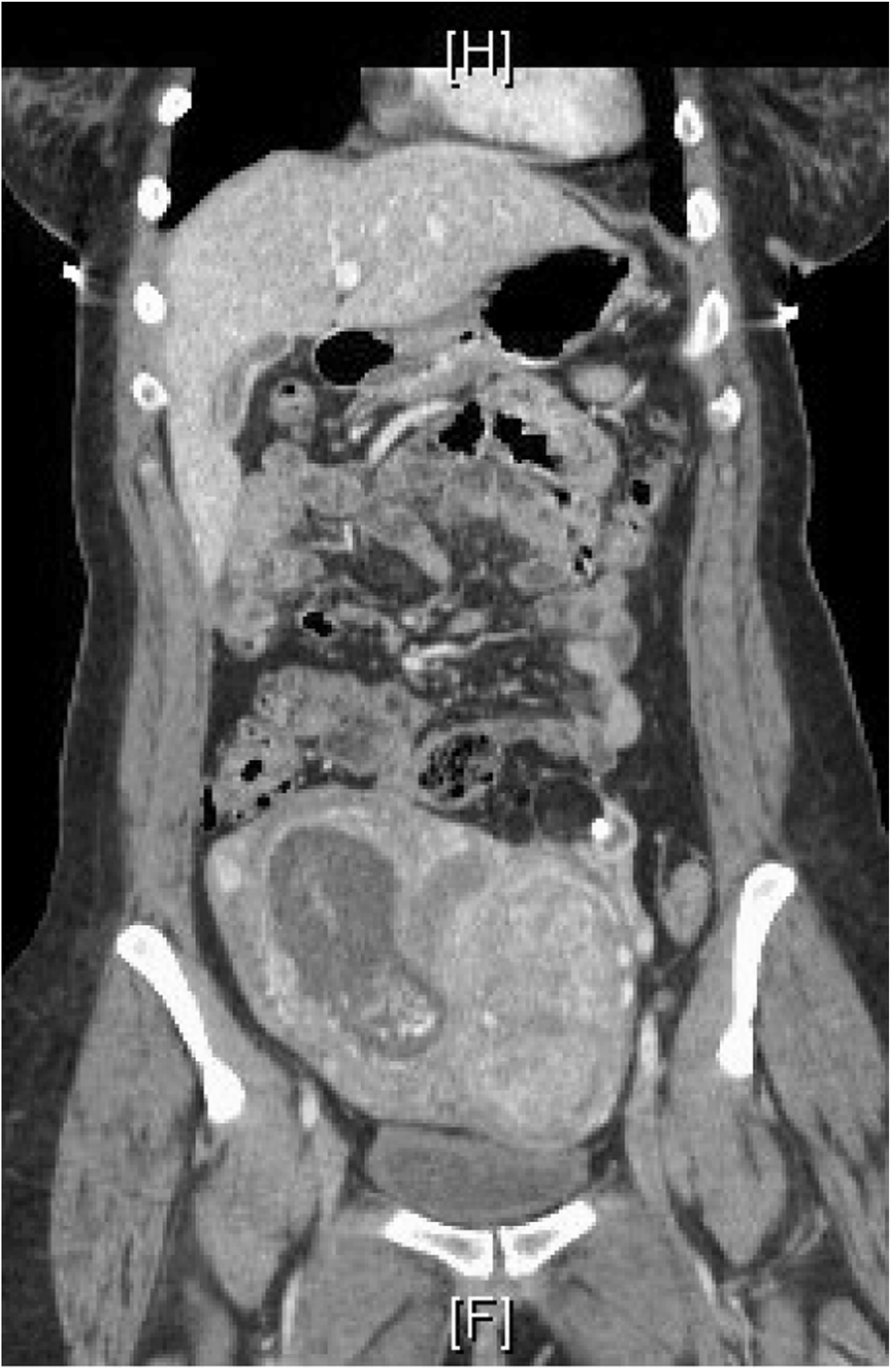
Coronal view – Abdominal CT scan showing a left sided ovarian teratoma and complete hydatidiform mole
